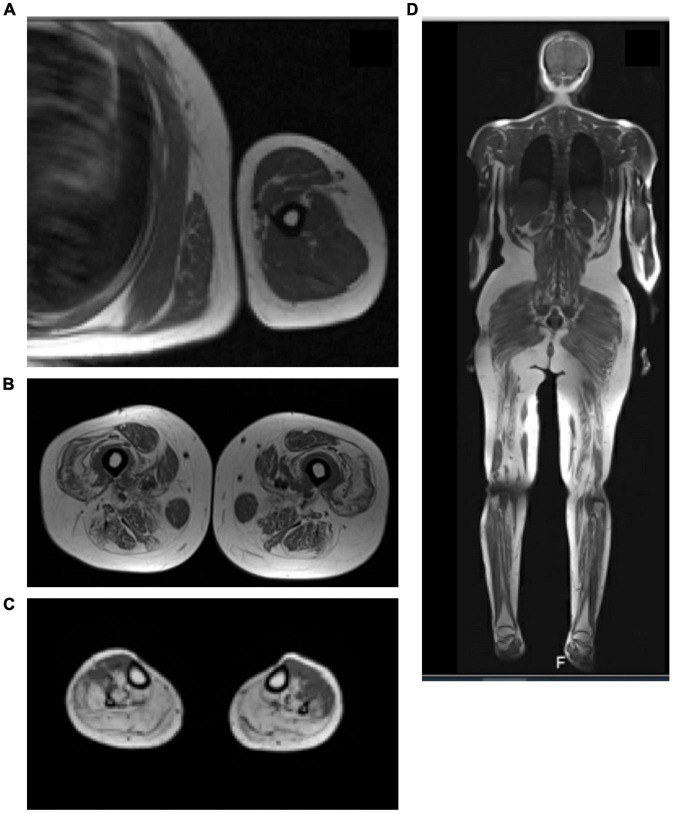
MRI of the leg with active inflammation. (A) MRI T1 of the upper limb showed the evidence of mild feathery muscle edematous changes involving the deltoid muscle and the biceps brachii muscle. (B) T1 MRI of the high muscle showed diffused fatty infiltration of the high muscle bilateral with sparing of the Sartorius muscle and relative sparing of the abductor. (C) T1 MRI of the legs showed significant fatty infiltration of the posterior and lateral compartments of the legs with relative sparing of the anterior compartment. (D) Whole body MRI scan (T1-weighted scan) showed diffused feathery edematous changes involve the thigh and leg muscle.
